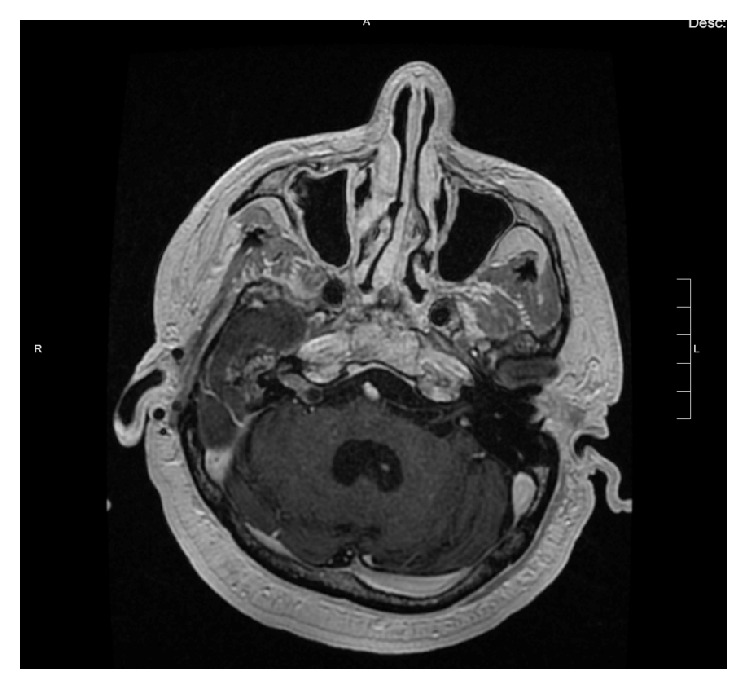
T1 weighted axial MRI demonstrating tumor growth in the cerebellopontine angle.
